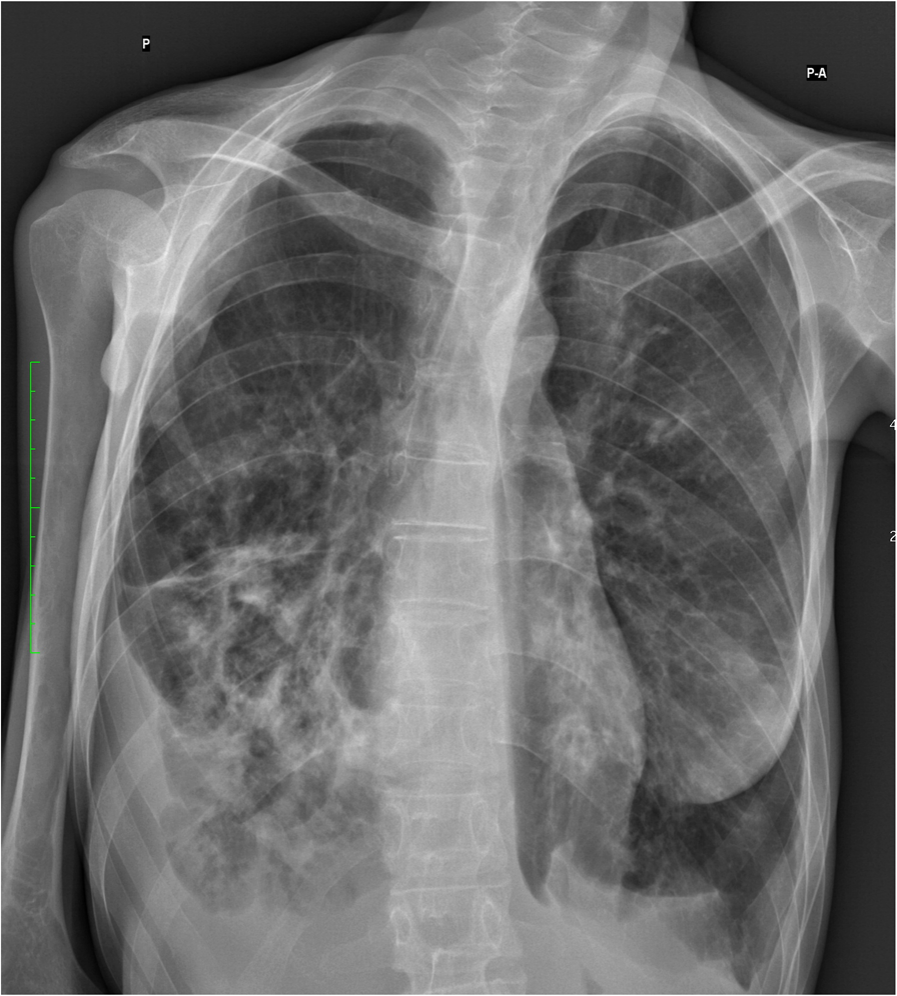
Chest X-ray on admission revealing multifocal consolidations mostly predominant in the right lung
